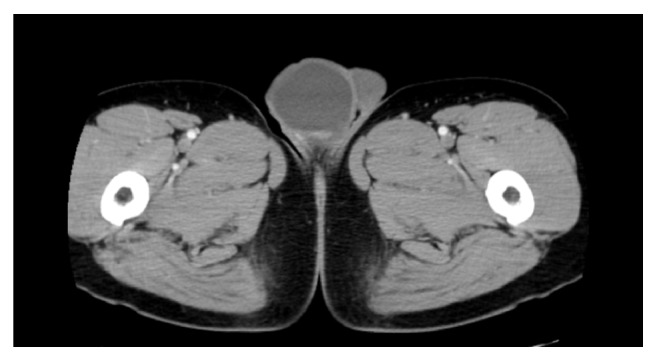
CT revealed a 48 x 48 x 42 mm cystic tumor. The wall of the cystic structure was thickened and enhanced with contrast medium.
